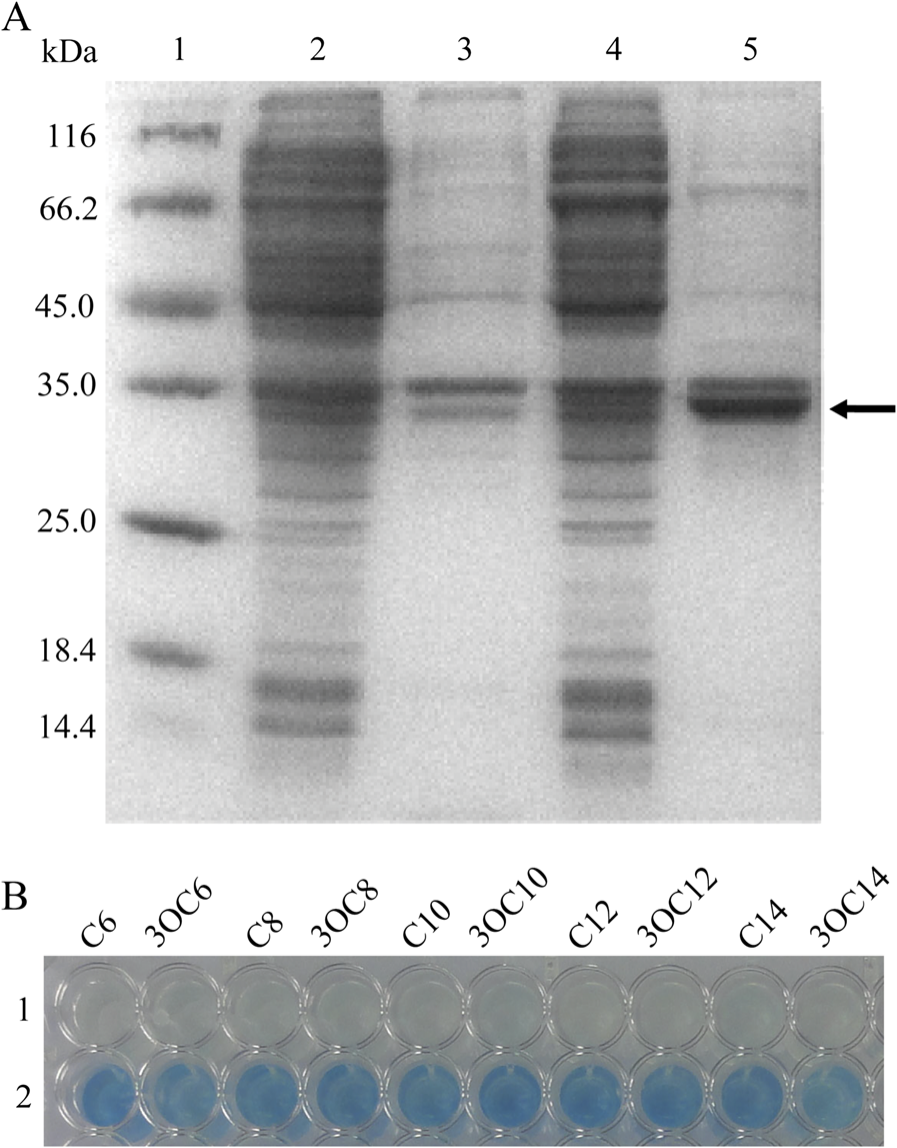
Expression of FiaL and detection of its QQ activity. (A). SDS-PAGE analysis of overexpressed FiaL. Lane 1, protein molecular weight marker (Fermentas SM0431); Lane 2, supernatant of *E. coli*/pET 24a(+) after ultrasonication; Lane 3, resuspended cell pellet of *E. coli*/pET 24a(+) after ultrasonication; Lane 4, supernatant of *E. coli*/pET 24a(+)/GL001211 after ultrasonication; Lane 5, resuspended cell pellet of *E. coli*/pET 24a(+)/GL001211 after ultrasonication (the arrow indicates the position of FiaL). (B). AHL-degrading activity of recombinant *E. coli* BL21(DE3). 1, supernatant of *E. coli*/pET 24a(+)/GL001211 after ultrasonication; 2, supernatant of *E. coli*/pET 24a(+) after ultrasonication.
